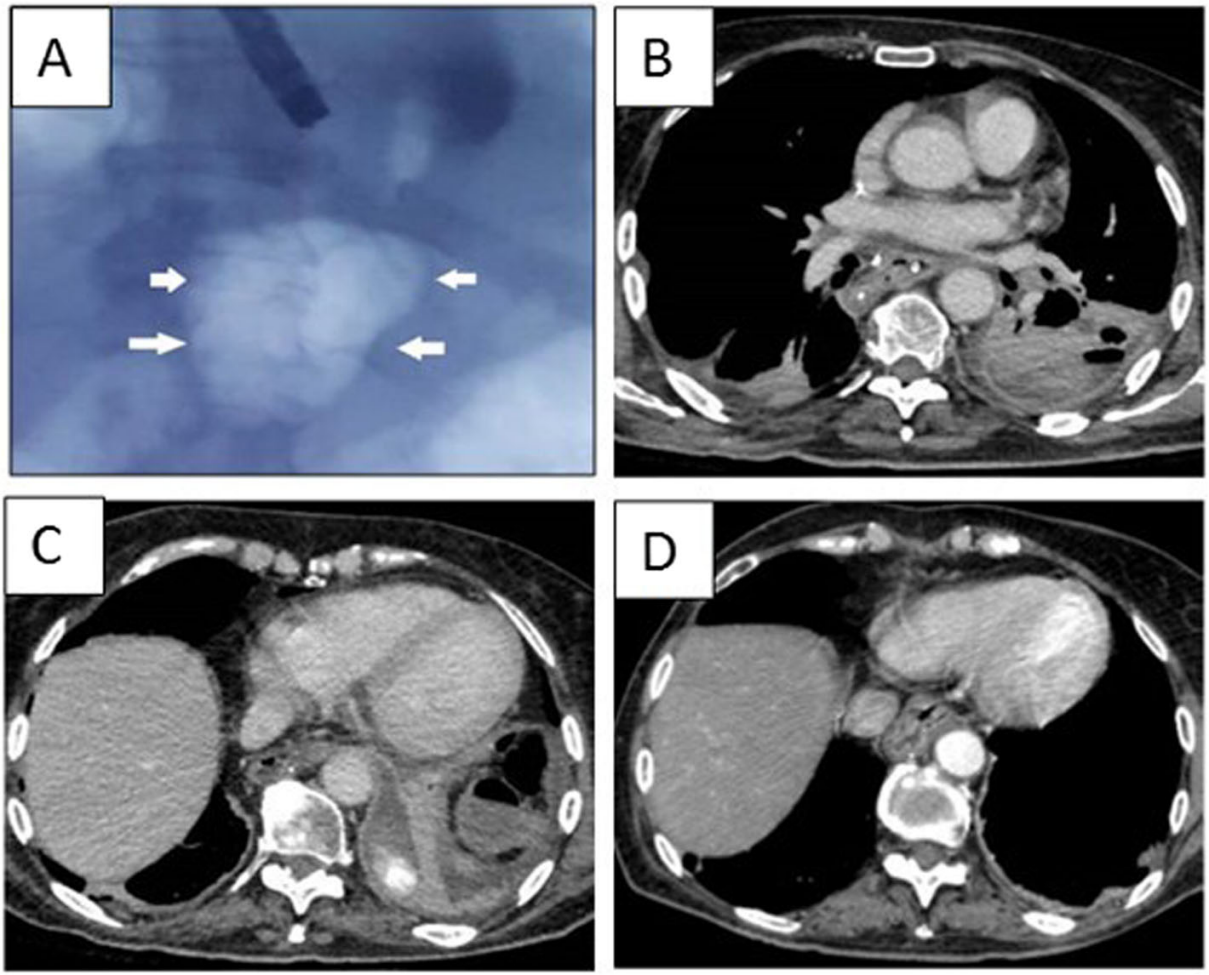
a Radiologic view of the paraesophageal abscess (white arrows) during endoscopic examiation. b, c CT scan showing a left pleuro-mediastinal abscess. d A 3-month follow-up.
